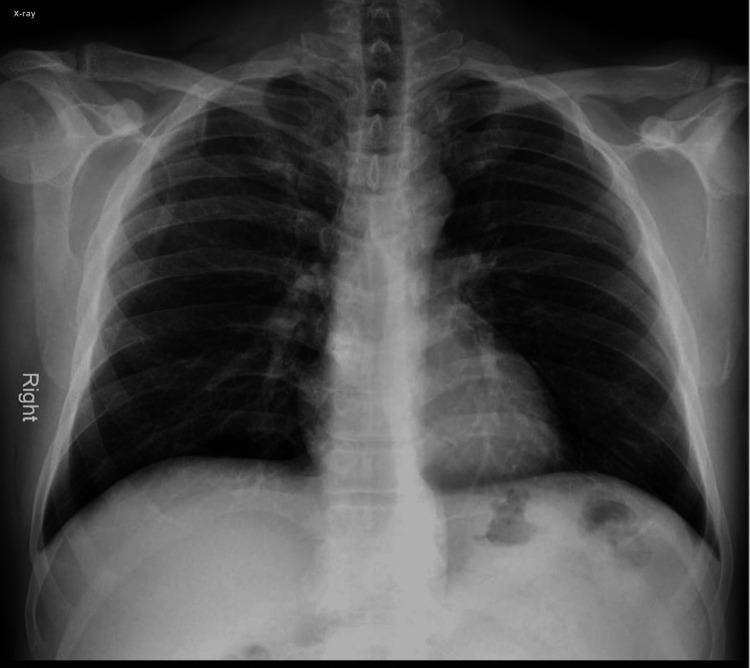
Mild COVID-19 pneumonia, but otherwise normal chest X-ray.
